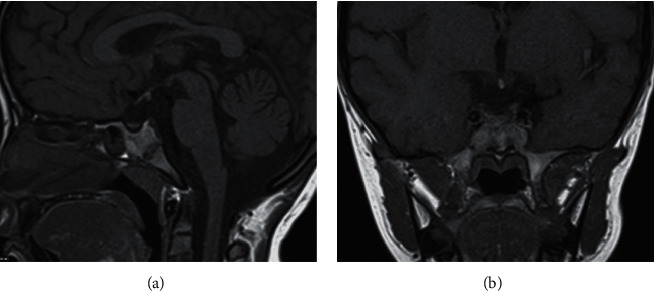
MRI image of the same child. (a) Sagittal T1WI image and (b) coronal T1WI image.
